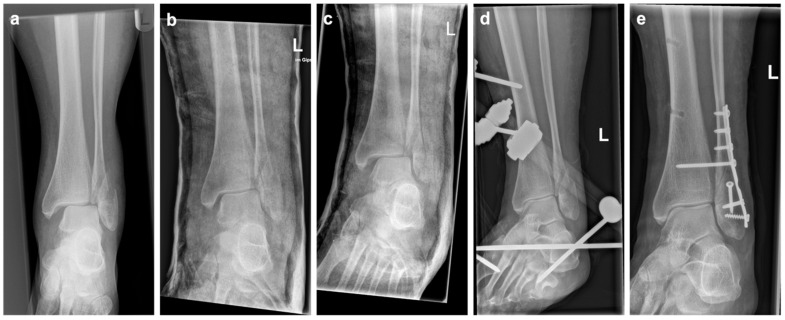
Example of a unimalleolar ankle fracture dislocation, with radiographs taken prereduction (a), postreduction with improved joint position in the cast (b), and after loss of reduction in the cast 3 days later (c). Subsequently, ExFix was applied (d) before definitive ORIF was performed (e).
